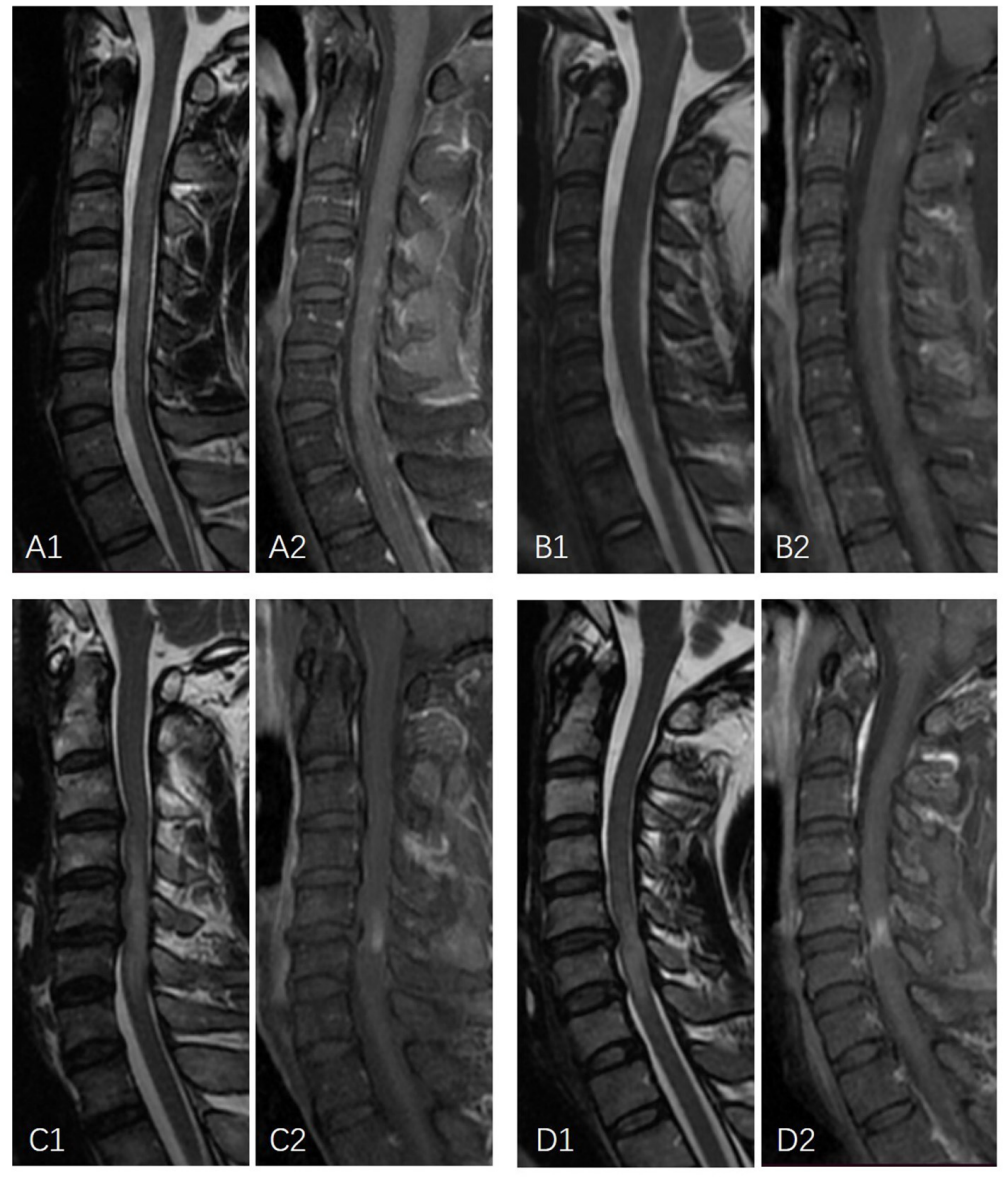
Examples of linear enhancement in NMOSD patients and longitudinally oriented flake-formed enhancement in long-segment DCM. (A1, A2) A 26-year-old male patient with NMOSD. Sagittal MRI demonstrates linear T2-weighted hyperintensity with discontinued linear gadolinium enhancement. (B1, B2) A 19-year-old female patient with NMOSD. Sagittal MRI demonstrates linear mildly elevated T2 signal intensity with linear gadolinium enhancement. (C1, C2) A 46-year-old male with T2-weighted hyperintensity extending over multiple vertebral segments with a longitudinally oriented enhancement flake-like appearance. (D1, D2) A 44-year-old male with T2-weighted hyperintensity extending over multiple vertebral segments with irregular flake-appearing enhancement with a longitudinally oriented long axis.
